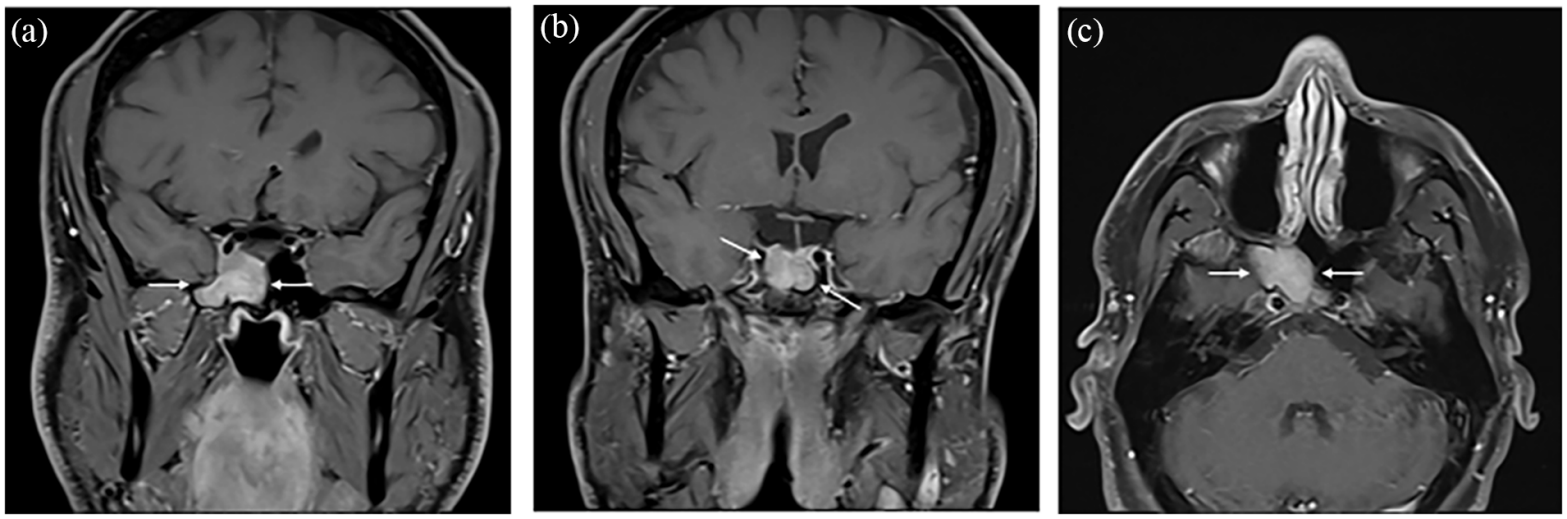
Preoperative MRI. Coronal (a, b) and axial (c) T1 post-gadolinium MRI showing enhancing mass involving the right inferolateral sella and extending into the right lateral recess of the sphenoid sinus as well as the cavernous sinus. MRI, magnetic resonance imaging.
